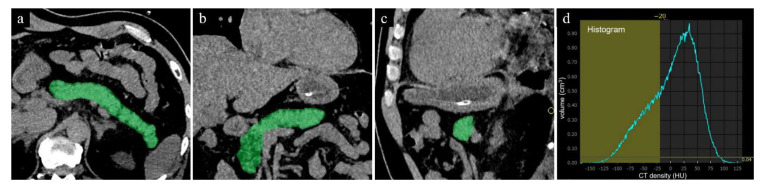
The measurement of the pancreatic fat volume on unenhanced CT. (a) axial, (b) coronal, (c) sagittal CT images. The pancreatic whole volume was measured in cm^3^ on unenhanced CT images using all slices, using an automated segmentation program installed in the workstation. (d) The pancreatic fat volume within the pancreas was calculated by means of a histogram analysis with local thresholding.
